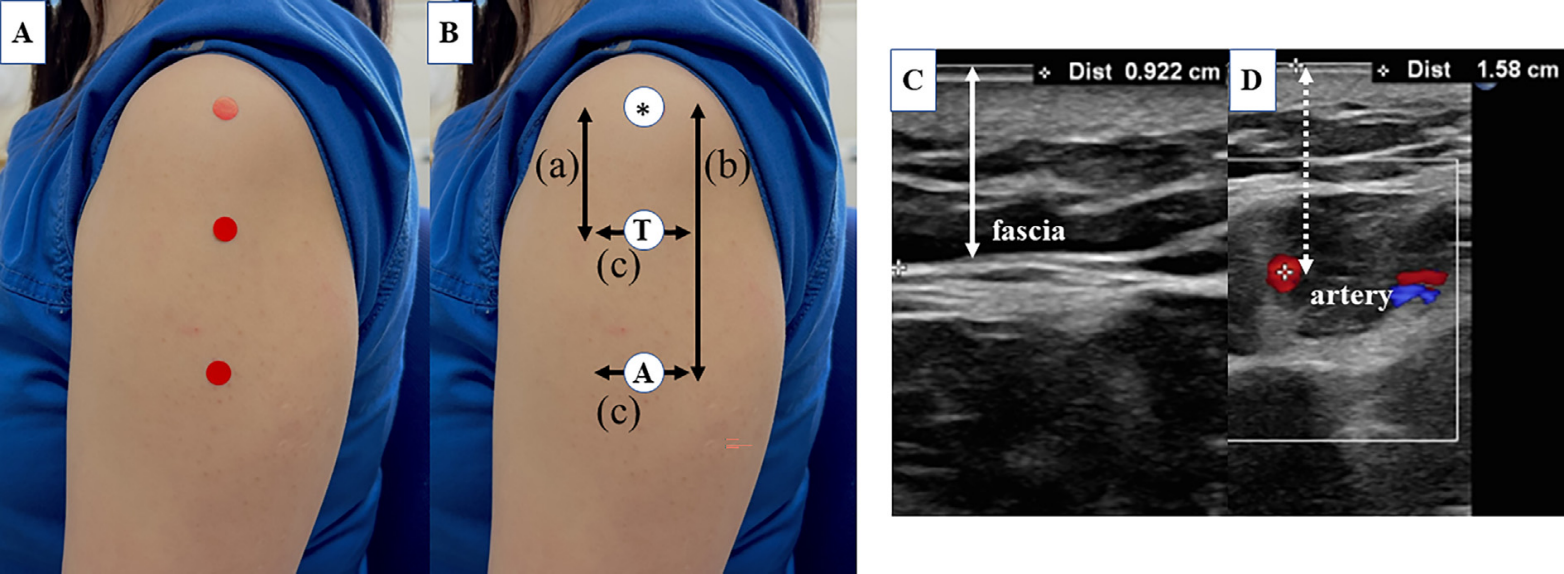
(A, B) The points that were investigated in this study are shown. * indicates the midportion of the mid-acromion lateral border. T represents the point at which the vaccine injection was performed using the three-finger breadth method. A represents the point of vaccine injection using the axillary method. (a) represents the length between * and the T point. (b) represents the length between * and the A point. (c) represents the length between the adjacent artery visible on ultrasound and the T or A point. (C, D) Ultrasound images of a male volunteer show the distance between the skin to the fascia at deltoid muscle (arrow) and the distance between the skin to the adjacent artery (dotted arrow) at the T point.
